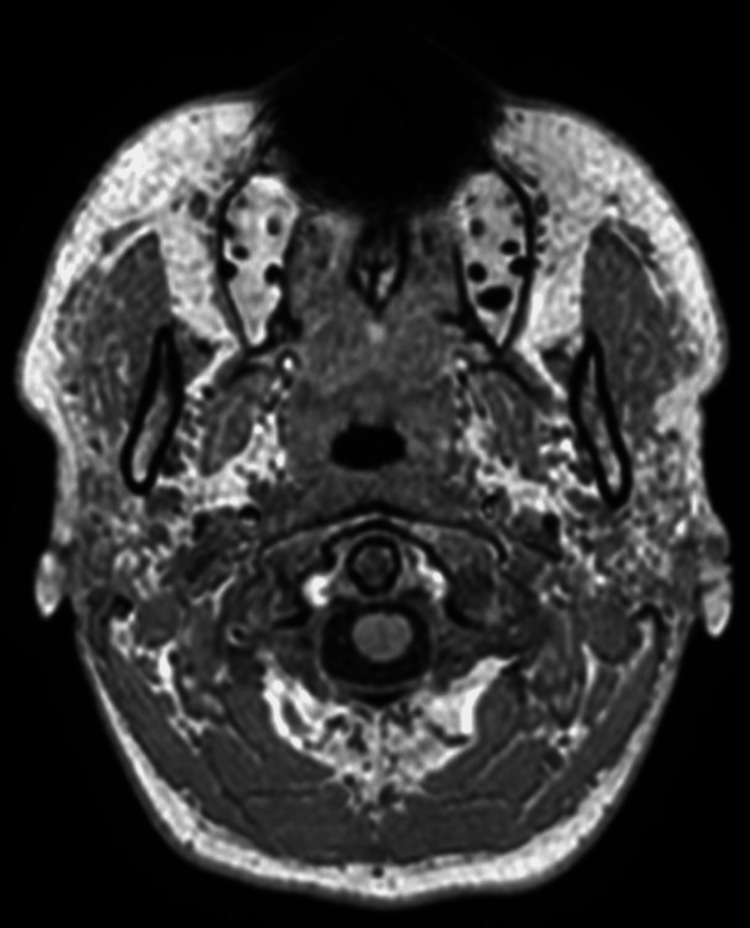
T1-weighted image depicts diffuse patch-like fat signals and residual glandular tissue with a nodule-like appearance, notably on the left parotid. Staging system for conventional imaging of the parotid glands proposed by Rao et al. [14], grade 3.
